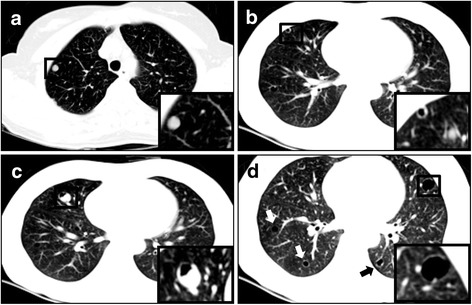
Chest CT reveals multiple cystic nodules. a A 6 mm-sized round nodule in the right upper apical segment. b A 1 cm-sized cystic lesion with a thin wall in both lungs’ parenchyma. c A 1 cm-sized cystic and well demarcated lesion with solid portion in the right middle lobe. d Multiple cystic nodules (arrows) with various sizes are in both lungs. CT: Computed tomography
